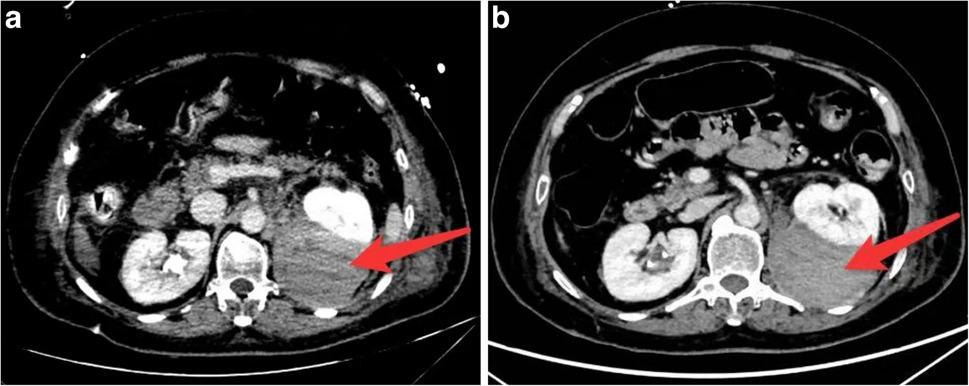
a CT image obtained on the first day after interventional surgery (hospital day 6), demonstrating a perirenal hematoma (red arrow). b CT image obtained on the 7th day after interventional surgery (hospital day 12), demonstrating a perirenal hematoma (red arrow)
